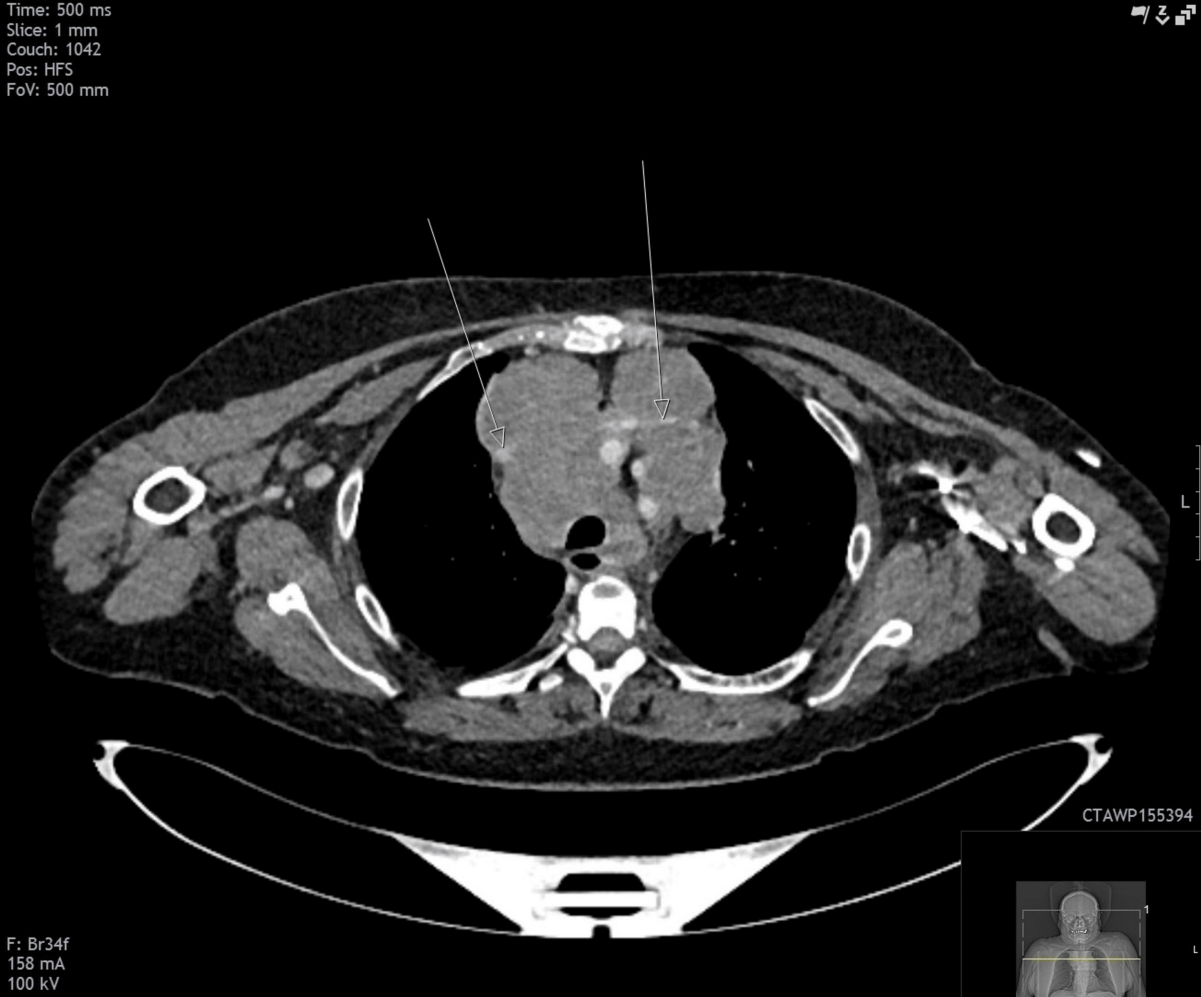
Axial CT demonstrating obstruction of bilateral brachiocephalic veins (labeled by the arrows)
